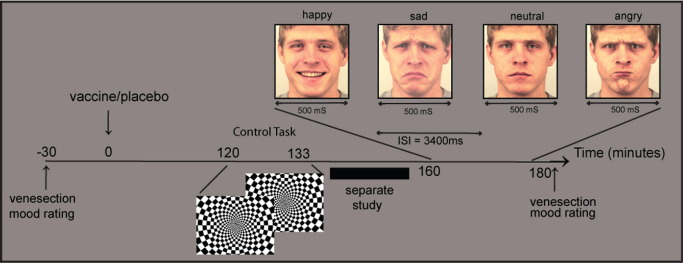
Study timeline. Participants completed mood rating questionnaires (Profile of Mood States [POMS]) and underwent venesection, then randomly received *Salmonella typhi* capsular polysaccharide vaccination (Typhim Vi, Aventis Pasteur MSD, Berkshire, United Kingdom) or normal saline placebo injection on two separate occasions 1 week apart. Two hours after injection, participants completed a flashing checkerboard control task, then an implicit facial emotion recognition task during functional magnetic resonance imaging. The POMS and venesection were repeated at 3 hours. Facial expressions reproduced with permission from the Section of Psychology, Department of Clinical Neuroscience, Karolinska Institutet, Stockholm, Sweden (23).
